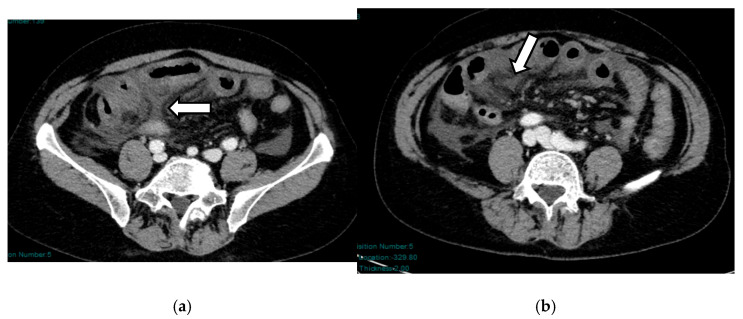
AMVI in a 64-year-old woman: axial contrast-enhanced CT scans show markedly thickened bowel walls of most of the ileal loops in the right iliac fossa and in the meso-hypogastric region, with reduced wall enhancing (a,b). Diffuse mesenteric thickening with free endoabdominal fluid (arrow).
